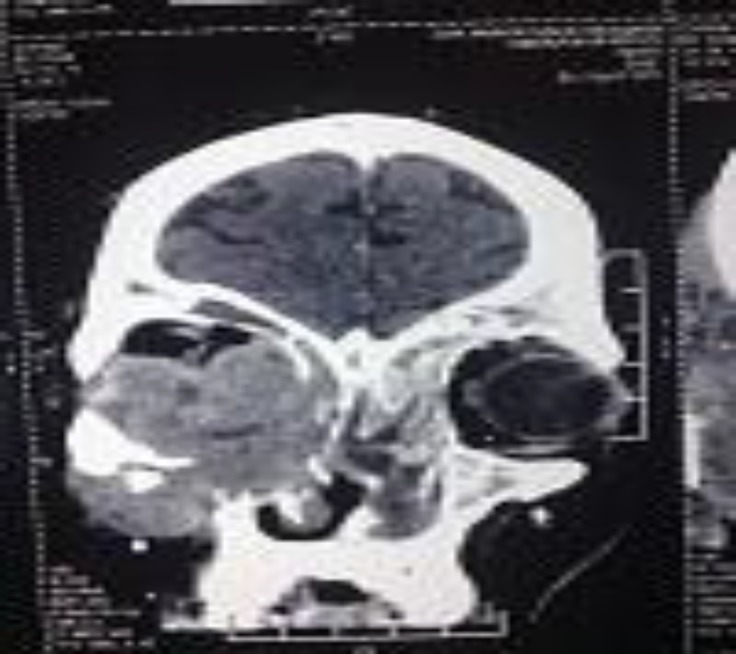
CT Scan PNS Coronal View showing intraocular involvement (Case 2
